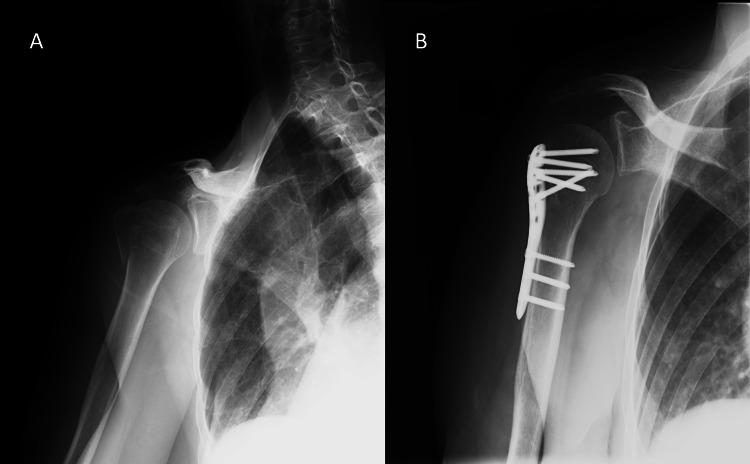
X-ray of the right shoulder A) before surgery B) at one month after surgery
